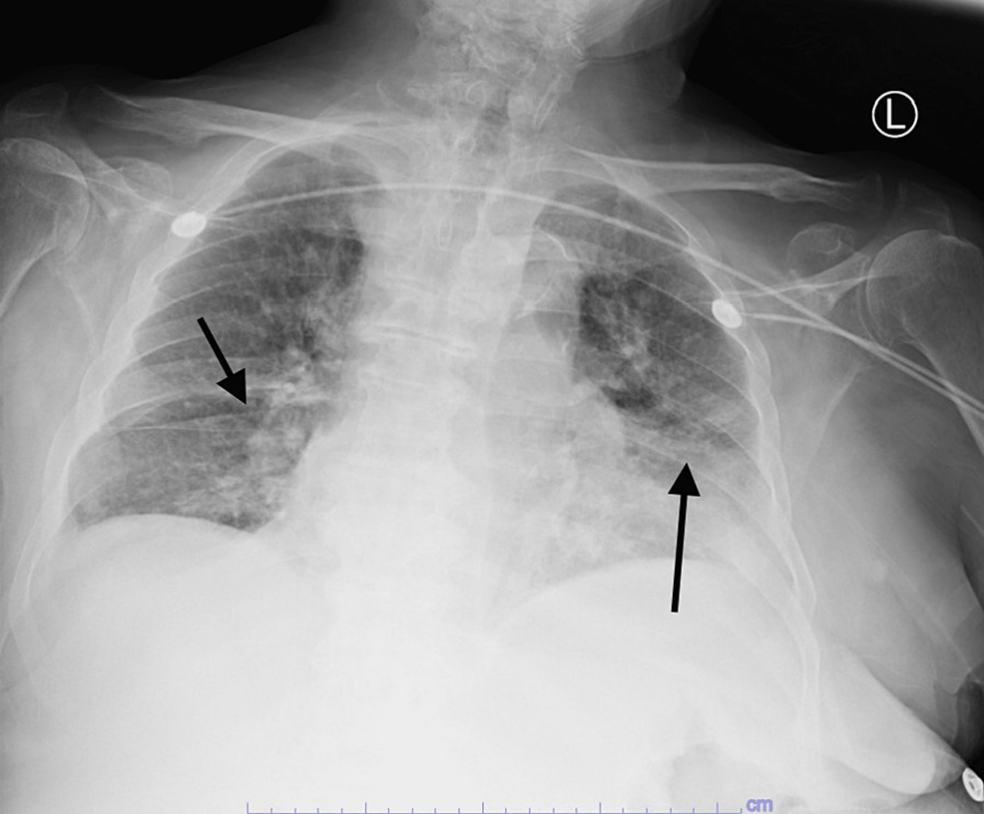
Chest X-ray Chest X-ray showing bilateral infiltrates suggestive of COVID-19 pneumonia
